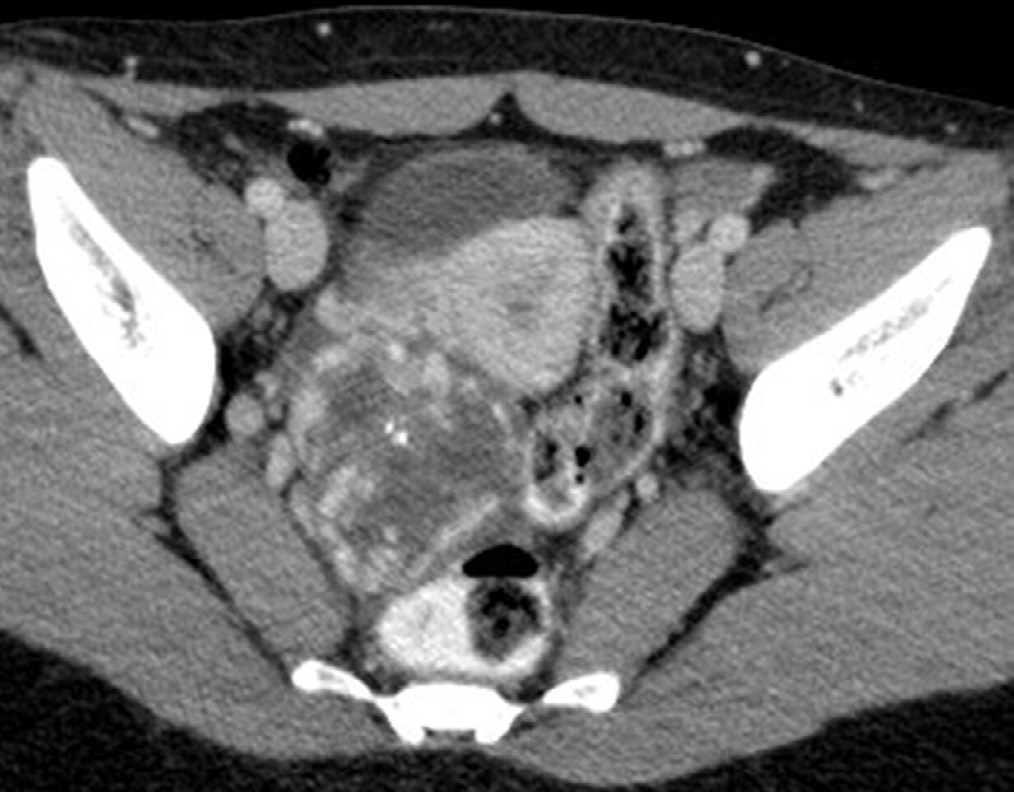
Axial contrast-enhanced CT shows a 6 cm mass in the right adnexal region with centrally located calcifications, a central cystic component and avid contrast peripheral enhancement.
